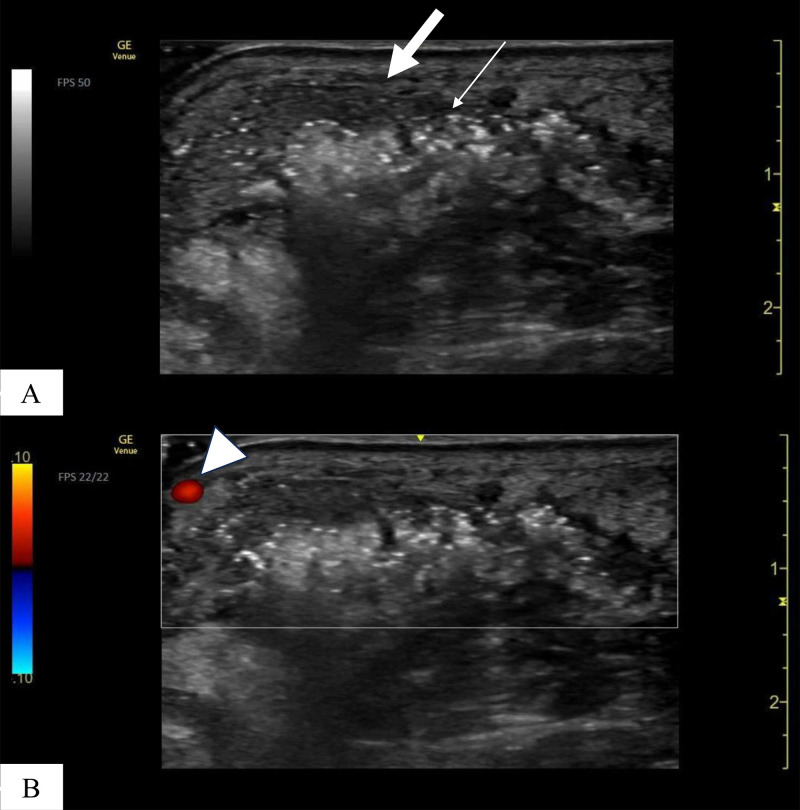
Ultrasound images of the injured site. (A) The subcutaneous layer demonstrated a thick hypoechoic region containing anechoic areas consistent with debris or fluid (thick white arrow), along with hyperechoic foci suggestive of intralesional gas (thin white arrow). (B) Colour Doppler imaging demonstrated the absence of intralesional flow within the subcutaneous abscess, while an adjacent blood vessel exhibited detectable Doppler flow (white arrowhead).
